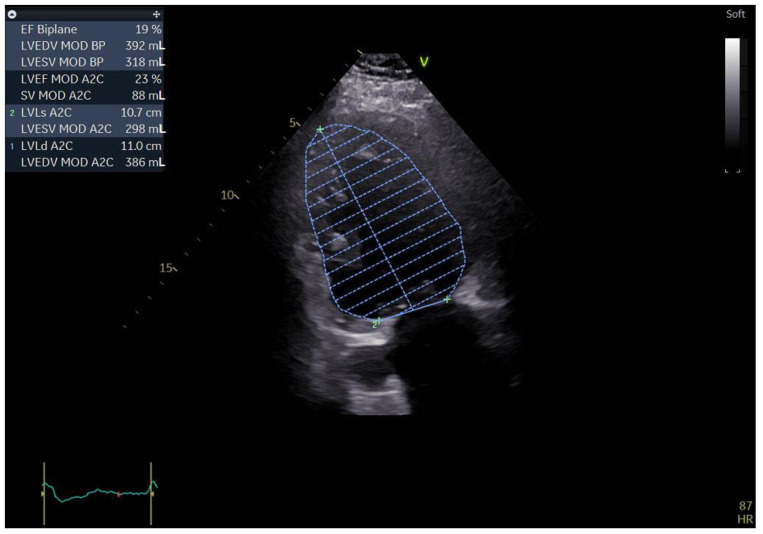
Two-dimensional echocardiography: apical two chamber view showing a severely dilated left ventricle along with a severely impaired LVEF; the top left of the picture showing the aforementioned measurements (biplane results).
